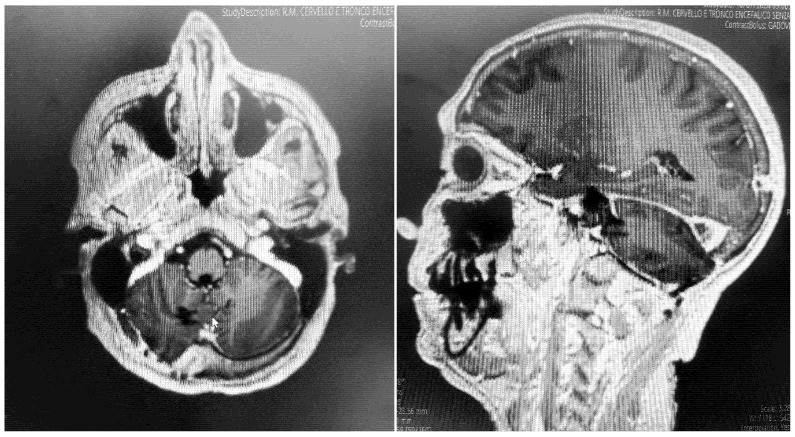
Post-contrast T1-weighted MRI at 18 months following resection. Axial and sagittal images at the level of the prior tumor demonstrate no nodular or mass-like enhancement to suggest residual or recurrent meningioma.
